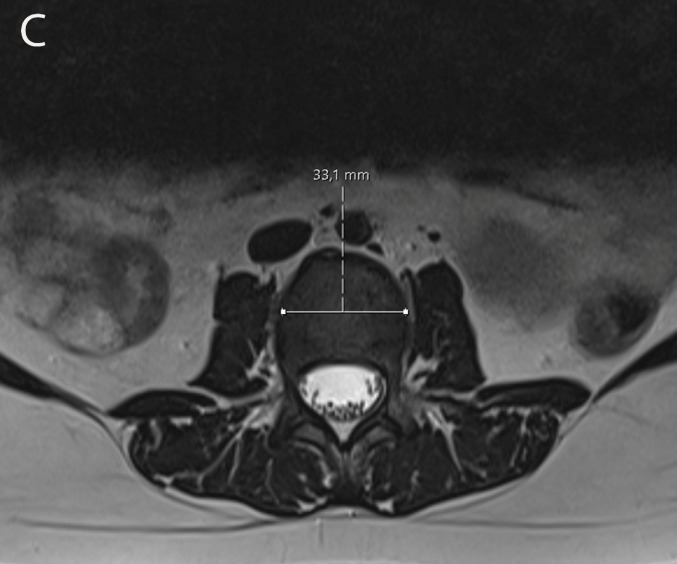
Vertebral MRI-based quantitative measurement methodology. (A) Signal intensities obtained by placing an ROI with a diameter of 10 mm in the L1-L4 vertebral bodies in T1-weighted midsagittal planes and reference measurements performed with cerebrospinal fluid at the L3 level. (B) Millimetric measurements of anteroposterior and longitudinal dimensions of vertebral bodies in the sagittal plane. (C) Measurements of transverse diameters passing through the equatorial plane of the vertebral body on axial T2-weighted sections. Volumetric calculations were carried out using these three orthogonal measurements
